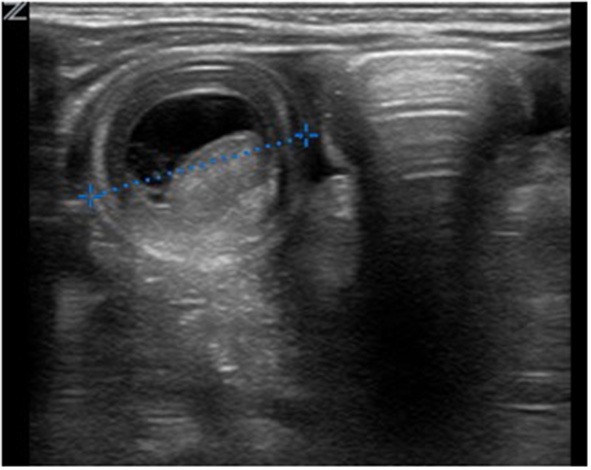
Sonography in the transverse plane of the right lower abdominal quadrant revealed the typical “target sign” of intussusception with a diameter of 27 mm, focal bowel wall thickening and fluid between the two bowel walls.
